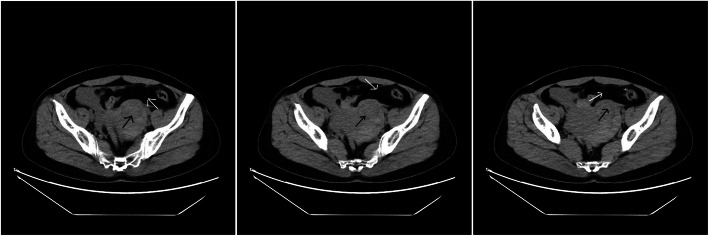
Preoperative CT scan of the abdomen with arrow mark showing adnexal mass (black) and bloody pelvic effusions (white)
